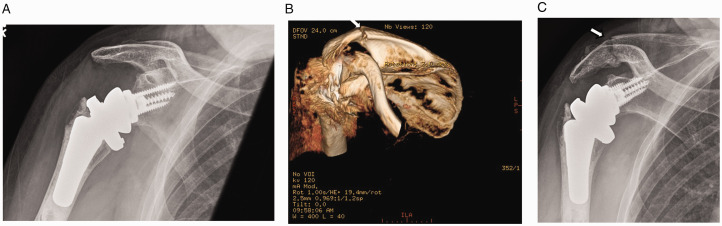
A, No obvious signs of stress fracture on plain radiographs in a 74 year-old male at 3 and ½ months post reverse arthroplasty. B, 1.2 mm helical CT scan images taken two weeks later showing healing fracture of the base of the acromion without significant displacement. C, Healing fracture of the base of the acromion now obvious on plain radiographs, taken two weeks after the CT scan and 4 weeks after the initial plain radiographs.
